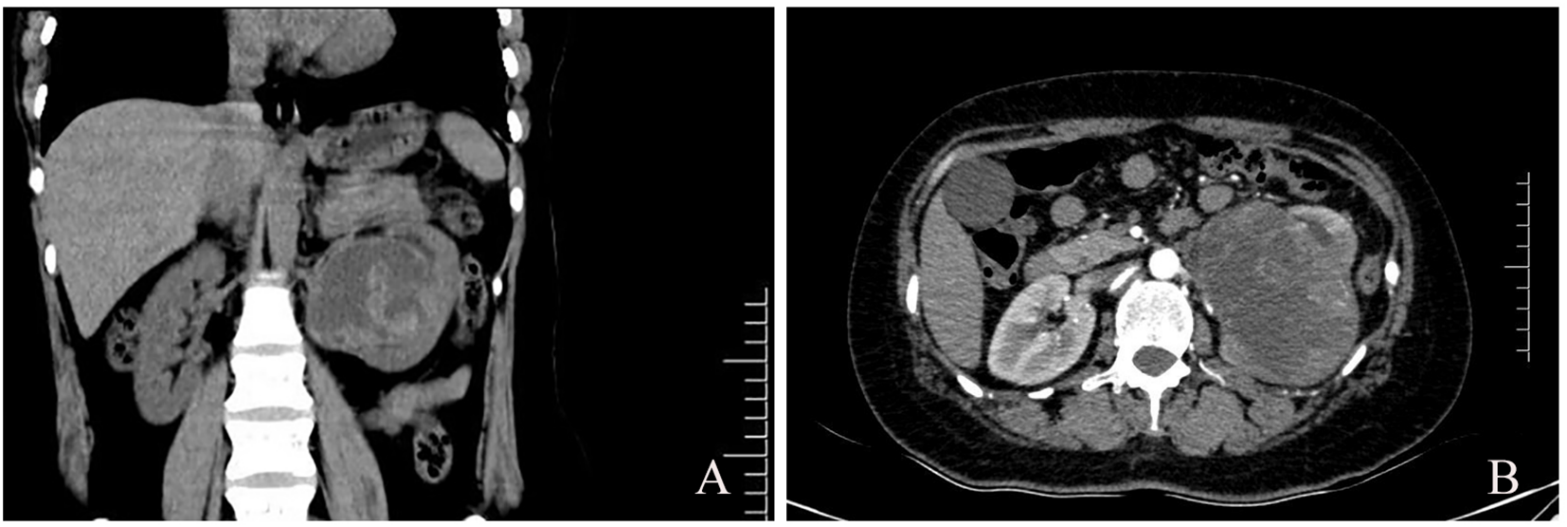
Abdominal CT. (A) Coronal view showing the tumor located in the middle of the kidney, occupying the position of the renal pelvis. (B) Axial view of contrast-enhanced CT demonstrating a large heterogeneously enhancing solid-cystic mass, measuring 9.32×7.46×6.24cm. No renal vein tumor thrombosis was observed.
